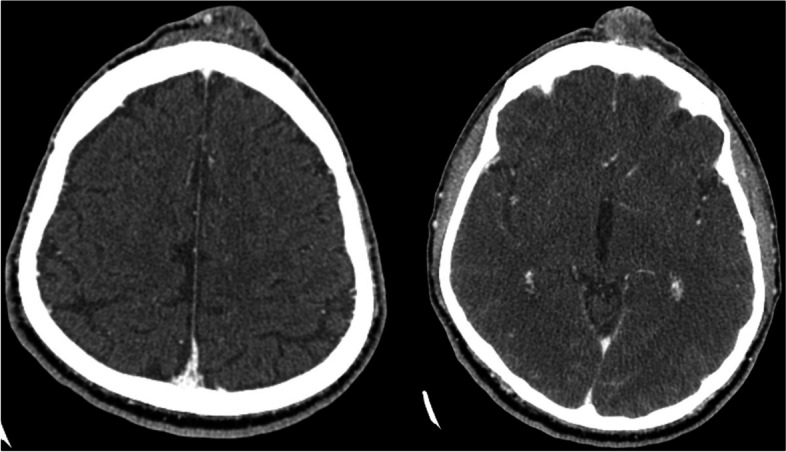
Imaging of a VM on the forehead of a 55-year-old patient using CT
